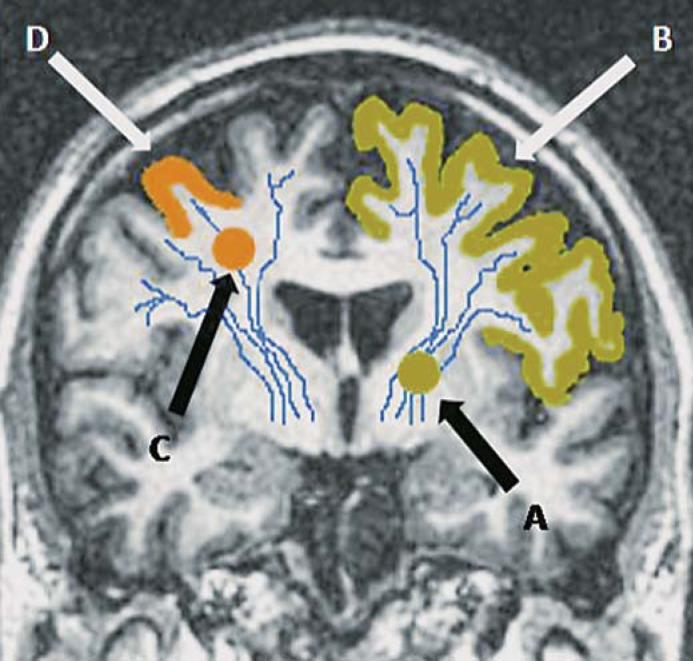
Coronal T_1_-weighted MRI brain to demonstrate how the site of a small subcortical (lacunar) infarct could influence clinical presentation. A small subcortical infarct lying in the left internal capsule, i.e. deep white matter (A), would cause functional disconnection of a large area of cortex (B, shaded). A peripheral small subcortical infarct lying close to cortex (C) would affect only a limited area of cortex (D, shaded), and could mimic a mild cortical stroke.
